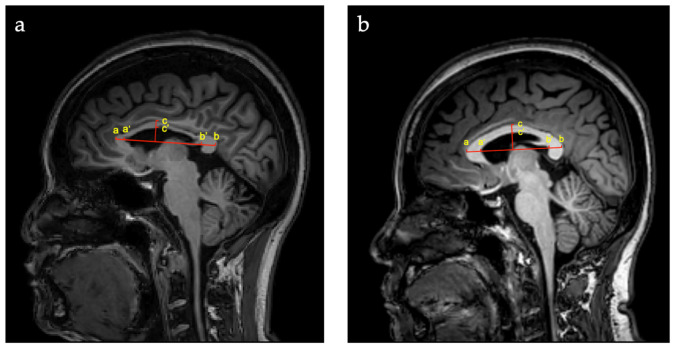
Measurement of the corpus callosum index (CCI) based on midsagittal T1-weighted images. Points a and a′, b and b′, and c and c′ were used to delineate the anterior, middle, and posterior segments of the corpus callosum, respectively. Each segment was then normalized to the maximum anteroposterior diameter of the corpus callosum (segment ab), yielding the CCI index. (a) Example of CCI measurement in a RRMS patient; (b) Example of CCI measurement in a control.
